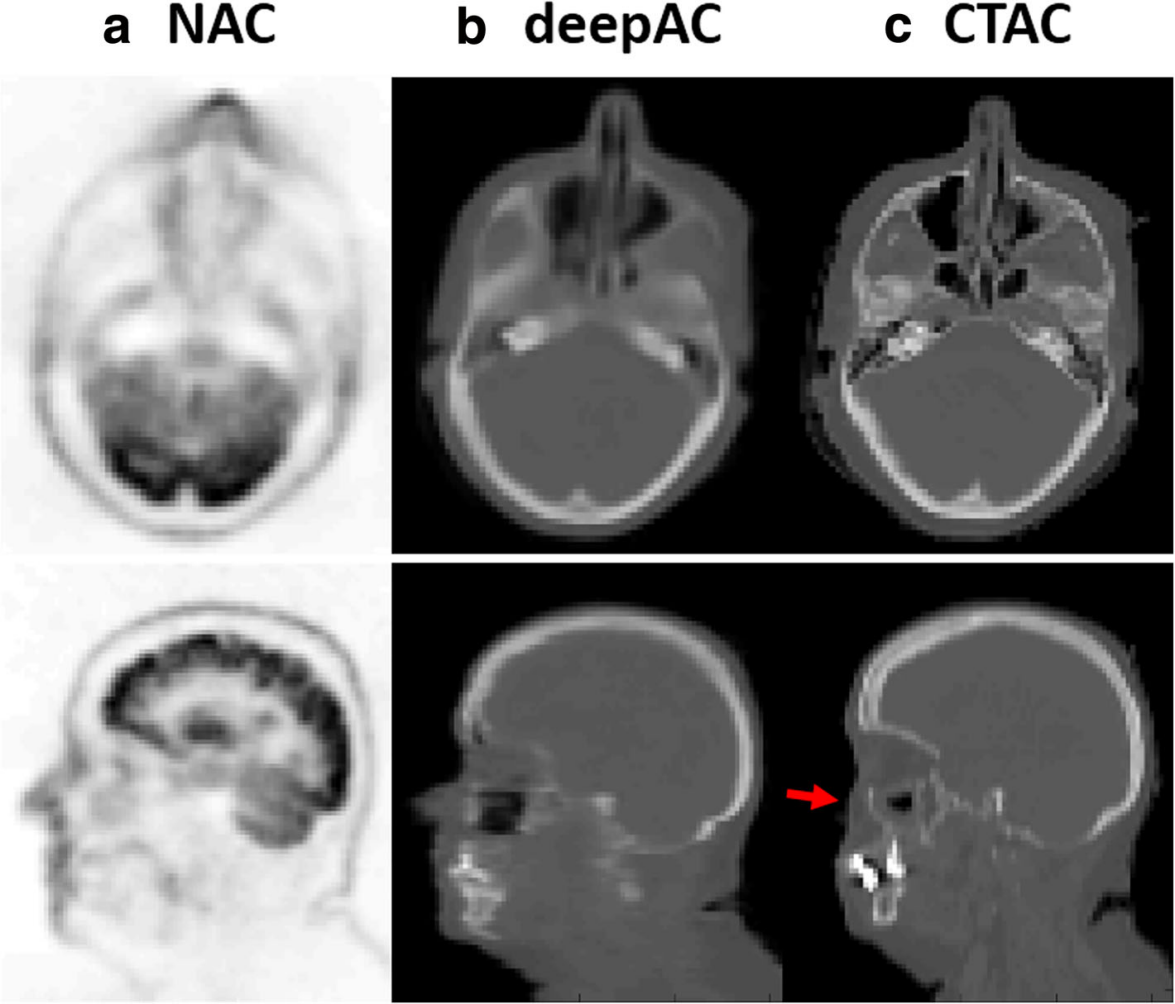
Example of pseudo-CT image from a non-compliant subject. Axial and sagittal slices from a the input NAC PET image, b the pseudo-CT generated using deepAC, and c the acquired CT. Note that there is a noticeable movement between PET and CT scans (red arrow). The generated pseudo-CT from deepAC is free from subject motion since it is directly obtained from PET data
